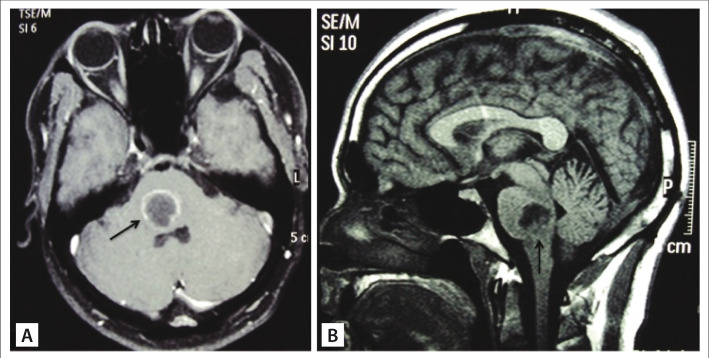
Magnetic resonance imaging showing low T1 signal, with ring enhancement at the dorsal pars of the pons (arrows). (A - axial view; B - sagittal view)
